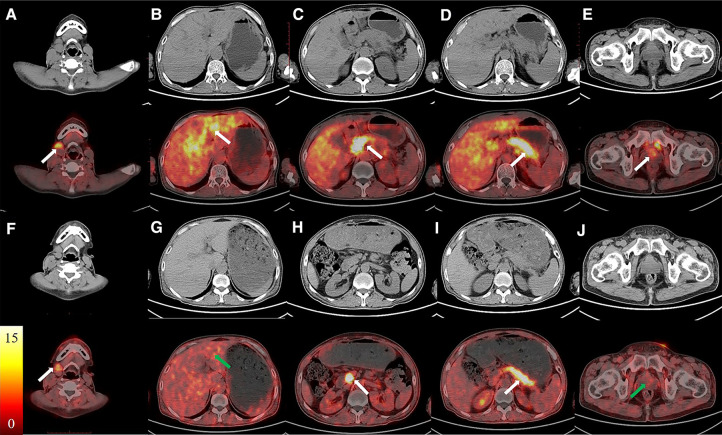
The images of ^68^Ga-FAPI PET/CT. The CT images were in the first/third rows, the fused PET/CT images were in second/fourth rows. The pre-treatment images (A–E, white arrows) showed elevated uptake of submandibular glands, intrahepatic bile ducts, pancreas, and prostate. The raised uptake of ^68^Ga-FAPI could still be observed in the submandibular glands and pancreas after treatment (F–J, white arrows).
